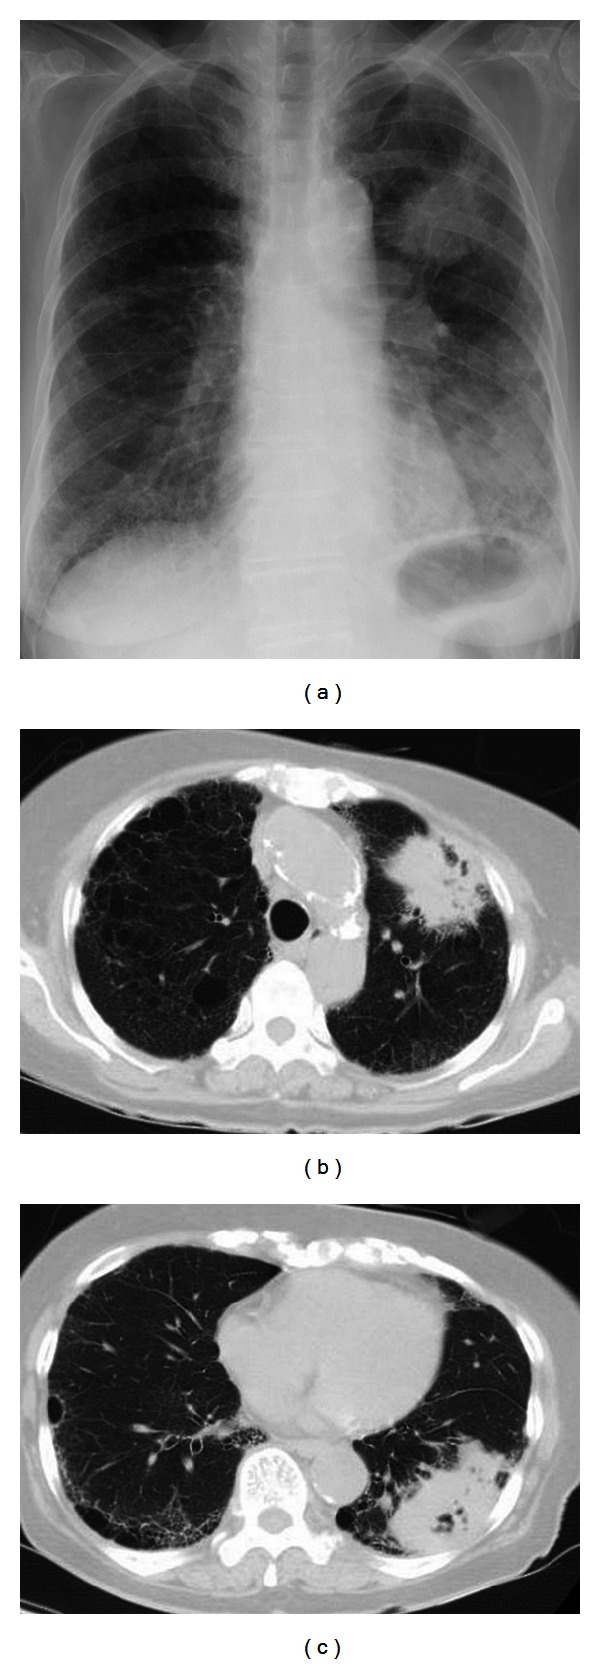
Chest radiography revealing a 5 cm mass in the upper lung field (a). Chest computed tomography (CT) revealing interstitial shadows in both lungs. Chest CT also revealed a 5.2 cm irregular mass with cavity and speculated margin in right S3 and a 7.6 cm irregular mass with cavity and air bronchogram sign, which are adjacent to the pleural walls ((b) and (c)).
